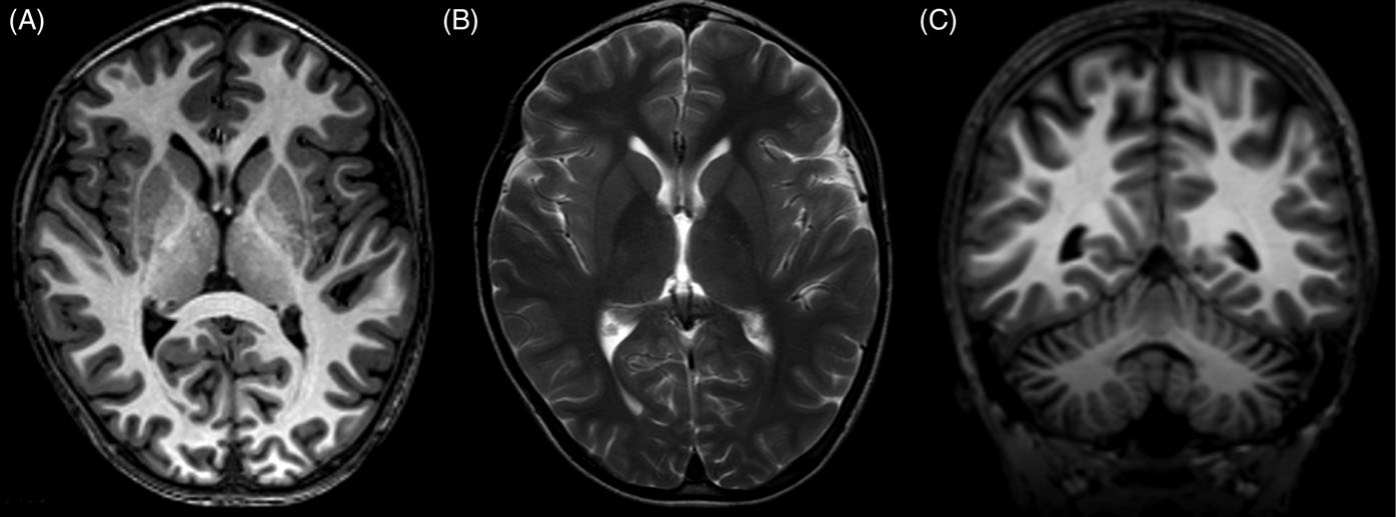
Clinical data: MRI images collected at the age of 9 years. MPRAGE (A) and T2‐weighted (B) axial images at the level of the basal ganglia and MPRAGE coronal images at the level of the middle cerebellar peduncles (C). Images were collected on a 3.00 T Achieva Philips Medical MRI scanner
